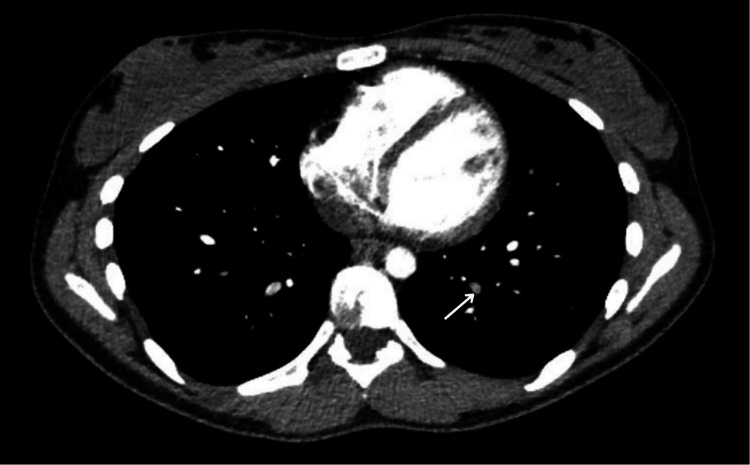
CT pulmonary angiography (CTPA) axial image demonstrating a filling defect within the segmental pulmonary vein branch draining the lower lobe of the left lung (arrow)
